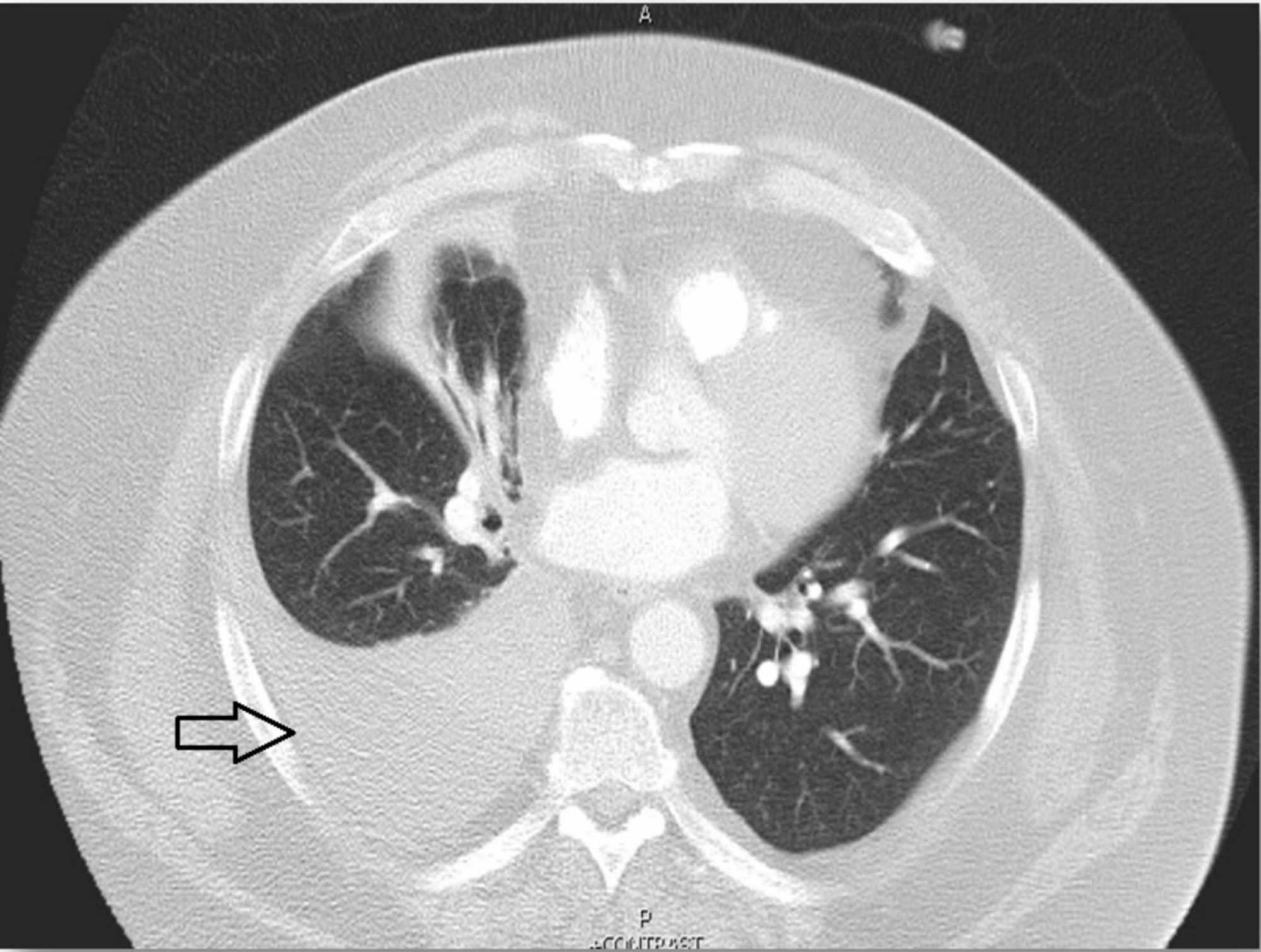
Computed tomography chest showing right-sided pleural effusion
